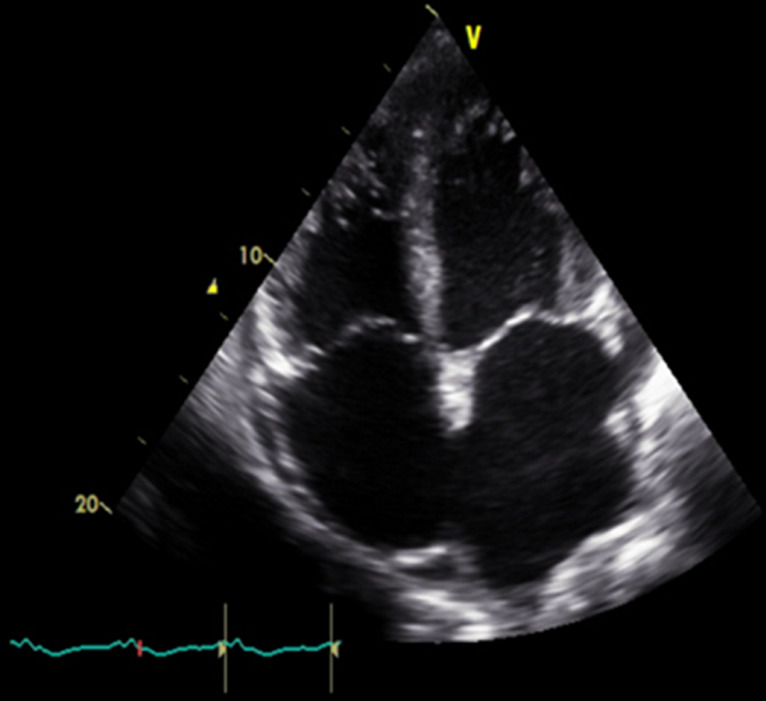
apical 4-chamber view, there is severe dilation of all the chambers and a small amount of pericardial effusion without tamponade physiology, and very subtle granular elements in LV chamber consistent with SEC
